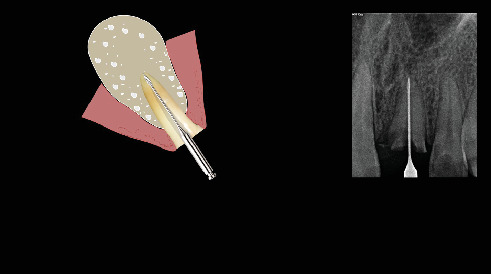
Reach the apex with gates glidden drill and confirm that on radiograph.
